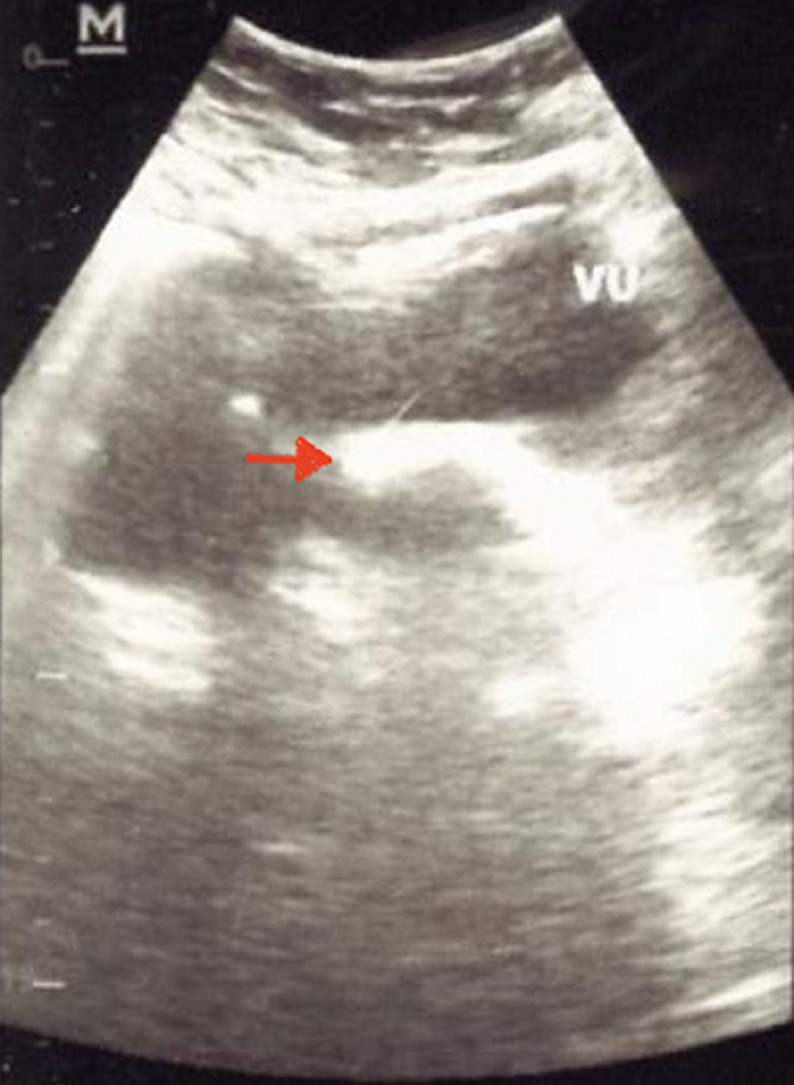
urologic ultrasonography showed a hyperechoic image in the bladder with sizes of 175 mm x 125 mm (red arrow)
